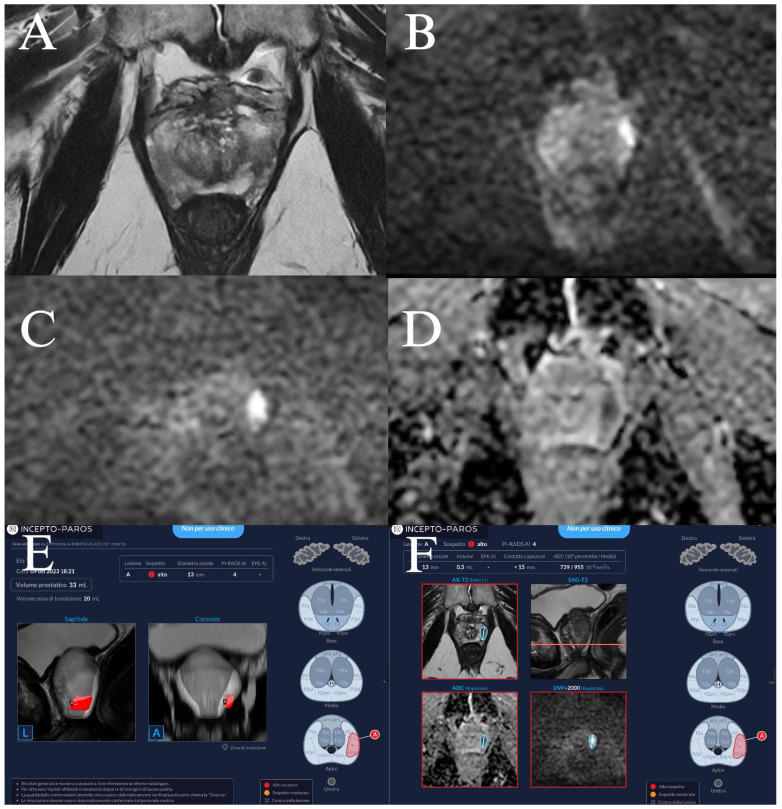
Example of a positive mpMRI prostate nodule in the left peripheral zone of the apex, displaying the original axial T2 (A), DWI with a b-value of 1000 (B), DWI with a b-value of 2000 (C), and ADC map (D). The PAROS report consists of a page displaying the measured prostate volume, and all identified lesions (E), both in 3D rendering and in axial slices of the prostate (red color is used to indicate PI-RADS 4 and 5 lesions). The next pages of the PAROS report (F) present each lesion in the axial T2 sequence, DWI, and ADC map with overlays. In this patient, the biopsy confirmed a csPCa with a Gleason score of 7.
